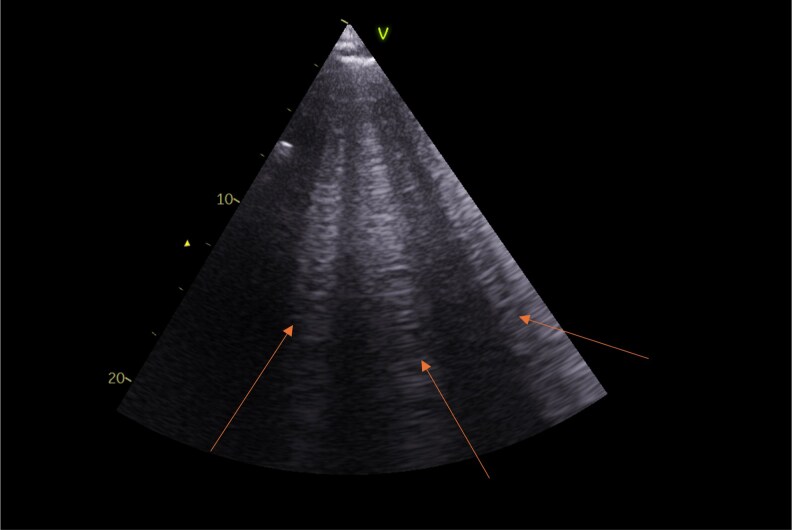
Lung ultrasound depicting B-lines (indicated by red arrows). A total of three B-lines are visible. Appropriate written consent was obtained from the patient for acquisition and use of this image.
